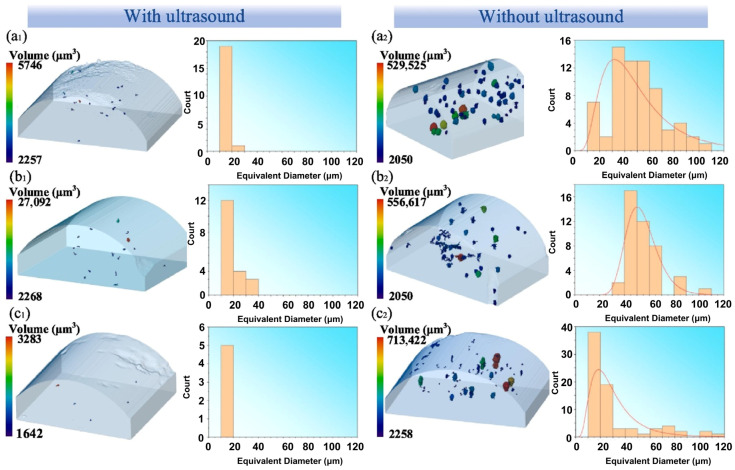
CT reconstructions of pore defects under three laser parameters: (a1,a2) Laser power of 1000 W with scanning speed of 10 mm/s; (b1,b2) laser power of 1400 W with scanning speed of 4 mm/s; (c1,c2) laser power of 1400 W with scanning speed of 10 mm/s [59]. The red line represents the fitted result.
